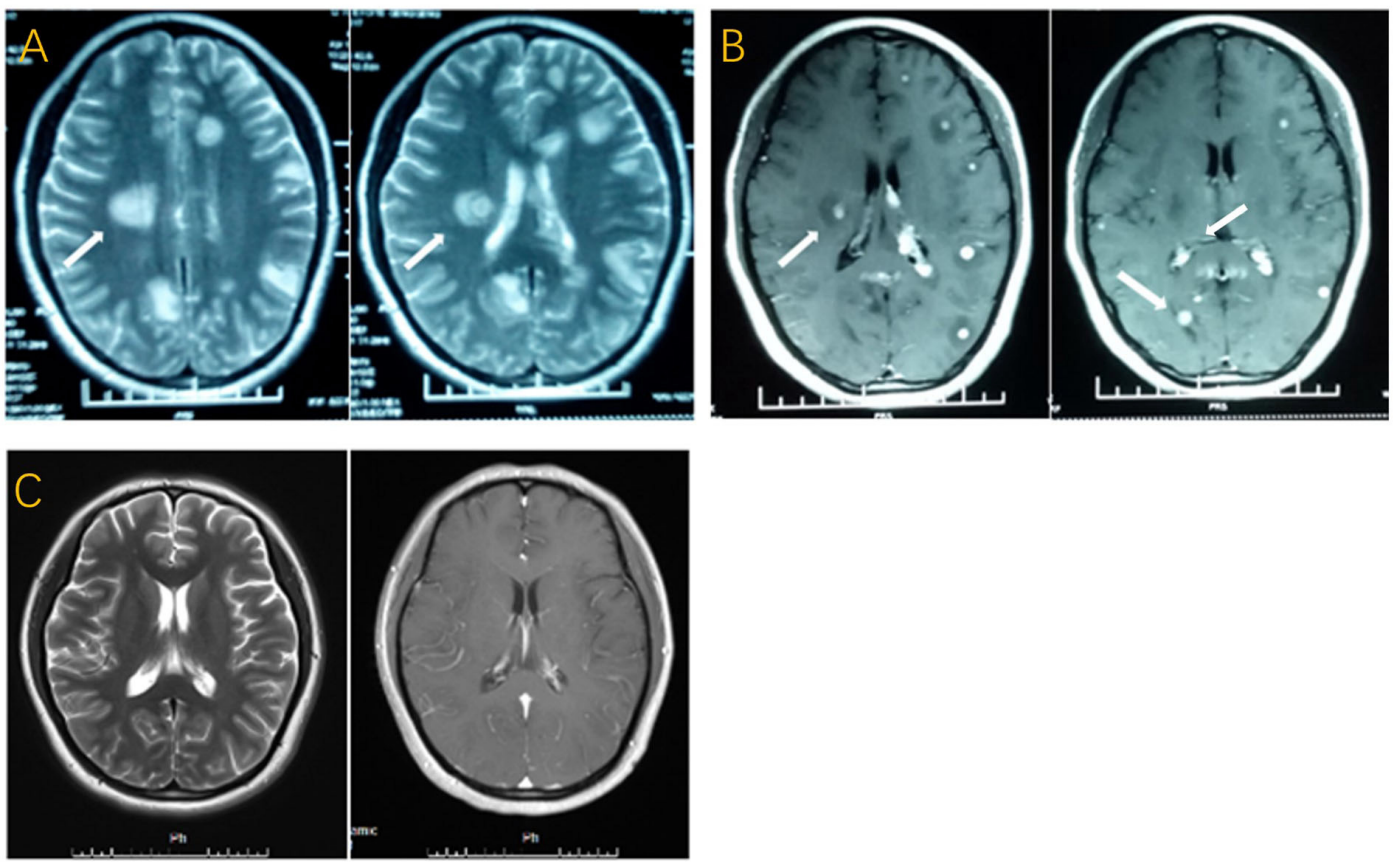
(A) T2-weighted image with multiple patchy high-intensity signals and “target sign”. (B) T1-weighted post-gadolinium contrast image with multiple nodular lesions and peripheral oedema. (C) T2-weighted image and T1-weighted post-gadolinium contrast image done three months later with approximate disappearance of the lesions.
